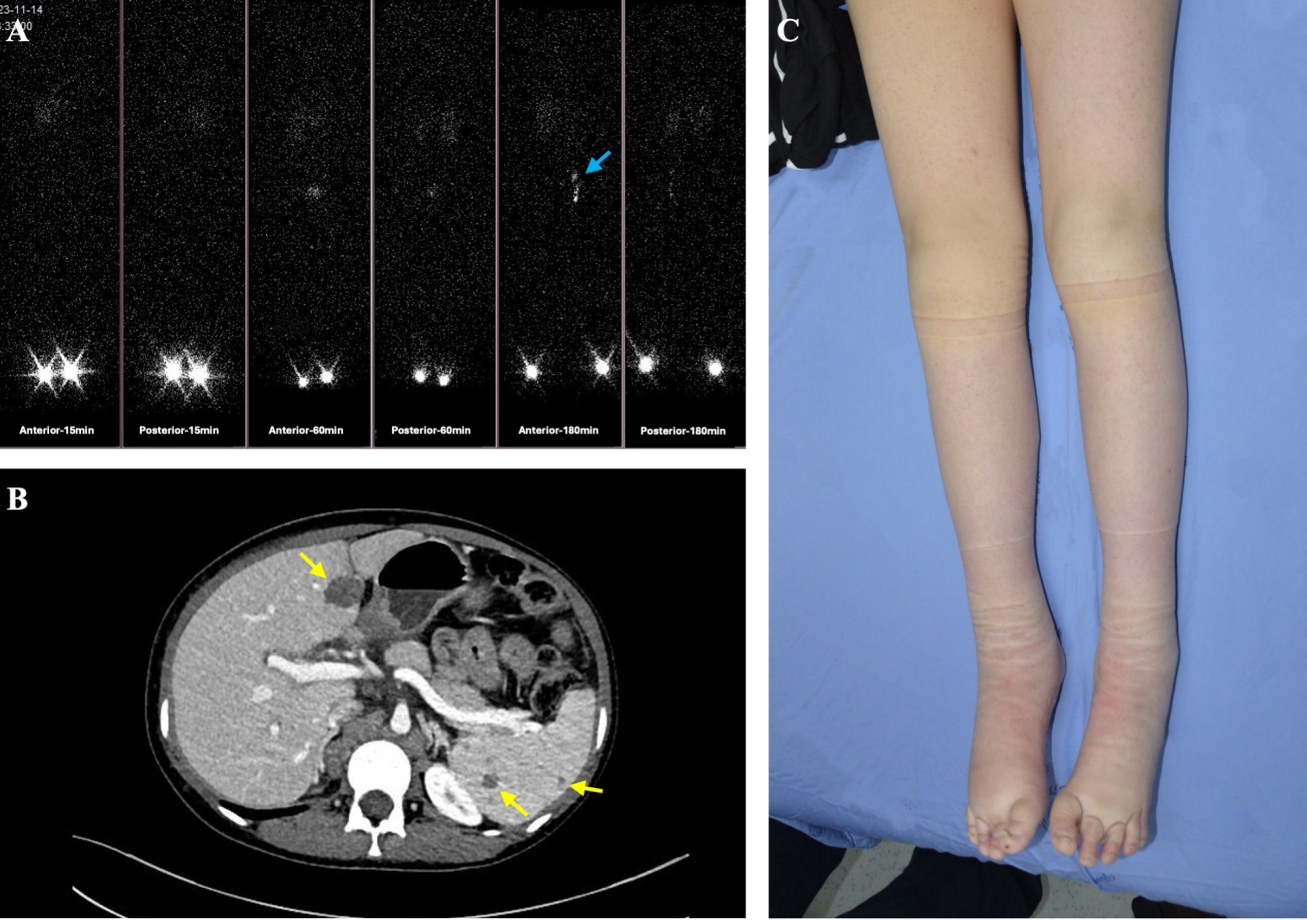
A female patient with generalized lymphatic anomaly (GLA) manifested multifocal cystic lesions in the liver and spleen, accompanied by bilateral lower extremity lymphedema. (A) Lymphoscintigraphy shows non-visualization of the right inguinal lymph nodes and delayed, faint visualization of the left inguinal lymph nodes at 15, 60, and 180 minutes post-injection in both feet (blue arrows). (B) Abdominal CT demonstrates multiple well-circumscribed, round hypodense lesions in the liver and spleen (yellow arrows). (C) Clinical photograph reveals extensive bilateral lower extremity swelling, more pronounced on the right, with localized skin thickening and textural changes.
